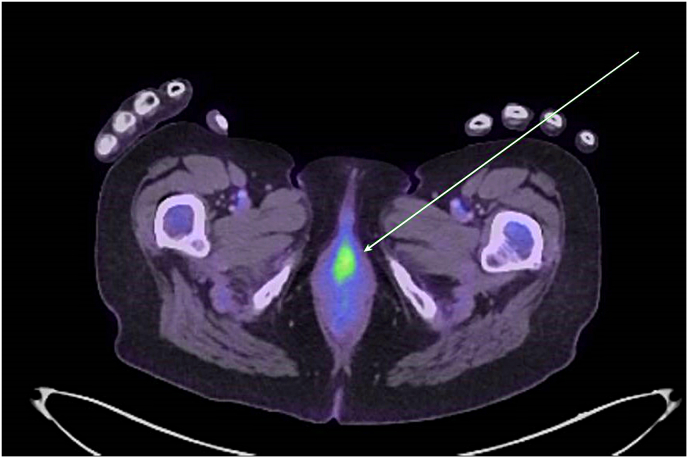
Whole body PET-CT. PET-CT performed post-operatively. FDG uptake around the resection site of the mid-urethra. This is consistent with residual disease/positive margins, extending over 20mm.
